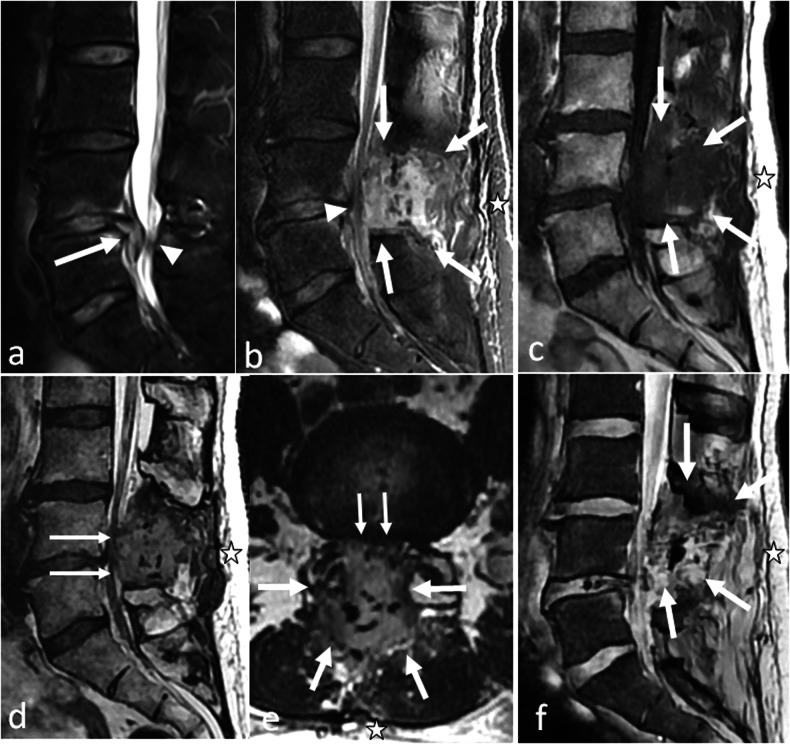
Cauda equina syndrome occurring three hours after a L4–L5 discectomy in 56 years old patient. MRI was performed in an emergency setting. On preoperative fat-suppressed sagittal T2-weighted images, a disc herniation issued from L4–L5 disk (arrow) was responsible for a dural sac compression with persistent cerebrospinal fluid (arrowhead) (a). On postoperative fat-suppressed sagittal T2-weighted images (b), sagittal T1-weighted images (c), sagittal (d) and axial (e) T2-weighted images, a heterogeneous collection lies in the operating bed (arrow), which results in a severe narrowing of the dural sac (arrowhead) with no persistent cerebrospinal fluid; this is best seen on axial images. Note that this is a deep haematoma without superficial component, thus no external bleeding (asterisks). Also, the dark elements that are visible within the haematoma on T2-weighted images (b, d, e) correspond to biological glue (f). On gradient echo T2-weighted images, there is no significant signal drop as this is an acute haematoma without hemosiderin. This latter sequence is not mandatory, and should not delay surgical re-intervention
